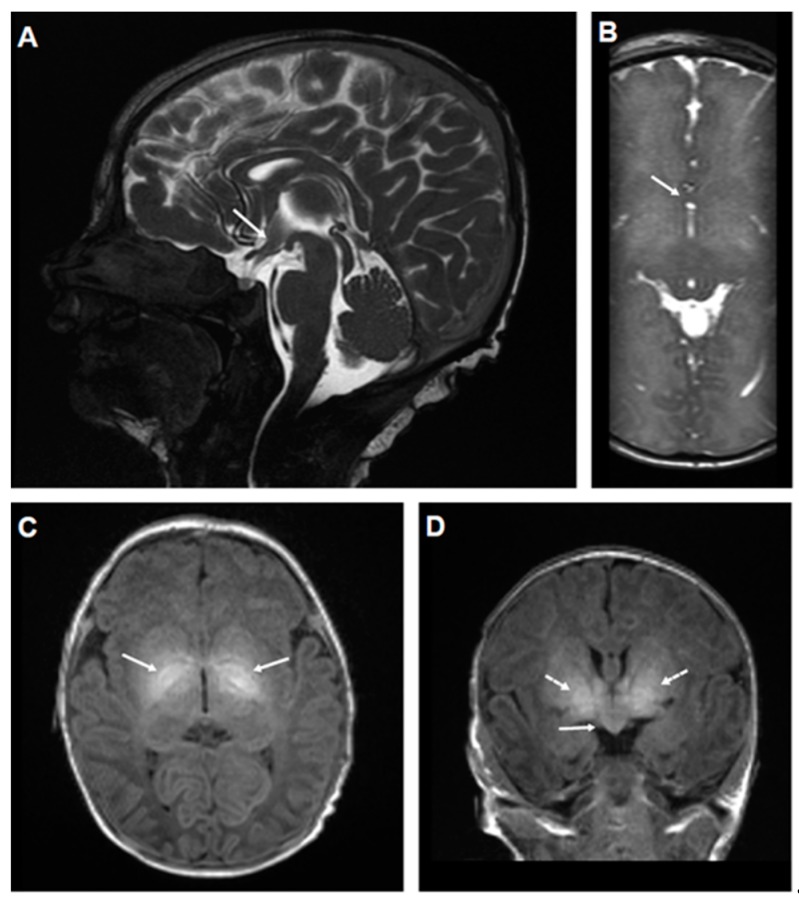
MRI performed on the proband. Neonatal MRI showing septopreoptic fusion abnormality and basal ganglia hyperintensity in a patient with KCNQ2 E140Q: (A) Sagittal T2 weighted steady state free procession (SSFP) image demonstrates abnormal tissue (arrow) extending through the anterior recess of the third ventricle in the septopreoptic area; (B) axial T2 SSFP image demonstrating interhemispheric fusion of brain parenchyma (arrow), corresponding to the tissue demonstrated in panel (A); (C) axial T1 image demonstrating abnormal hyperintense signal within the basal ganglia (arrows), especially the globus pallidus; (D) coronal T1 image showing both abnormal signal in the basal ganglia as in (C) (dotted arrows), and the midline fusion of brain parenchyma in the preoptic area (solid arrow).
